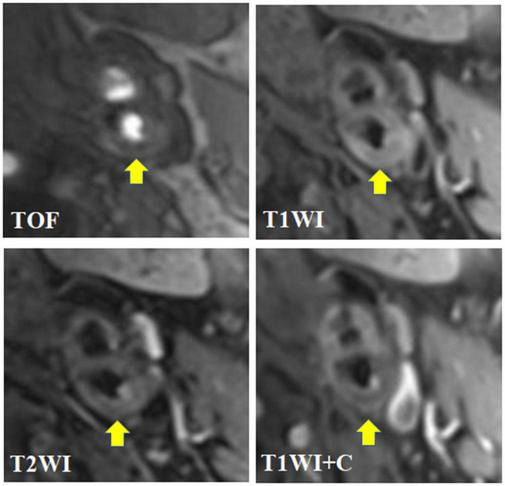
A 62-year-old male presented with dizziness and numbness of right limbs for more than 1 month. The transverse axial TOF-MRA showed moderate stenosis in the initial segment of the left internal carotid artery with the formation of centripetal plaques. The plaques were mainly slightly low signal with a small amount of high signal; HR-MRI transverse axial T1WI showed that the right internal carotid artery plaque was mainly slightly high signal, and slightly low signal shadow could be seen in it; HR-MRI transverse axis T2WI showed that the left internal carotid artery plaque was mainly slightly high signal, mixed with a small amount of low signal shadow, and the plaque surface showed irregular high signal; HR-MRI axial T1WI enhancement showed that the surface of left carotid artery plaque showed obvious interrupted linear enhancement, but no enhancement was found in the plaque; To sum up, the composition of plaques in the initial segment of the left internal carotid artery is complex, with visible lipid nuclei and calcification. The surface fiber cap rupture niche is formed with a small amount of bleeding (subacute), which belongs to AHA type VI, namely, vulnerable plaque.
